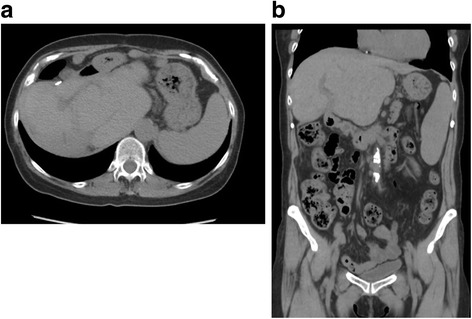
Computed tomography images of case 1. a and b No recurrence is noted
